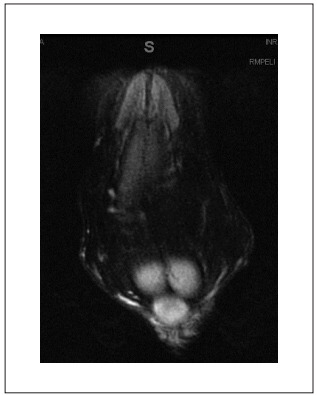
Magnetic resonance image (MRI) showing diffusely enhanced penile subcutaneous thickness and compressive effect of the mass over the corpora cavernosa and corpus spongiosum.
